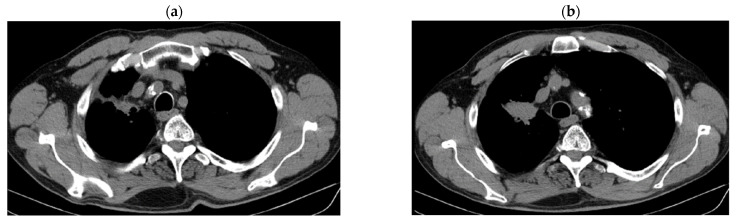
(a,b) Reevaluation CT from 02/11/2020 vs. CT from 04/2020.
